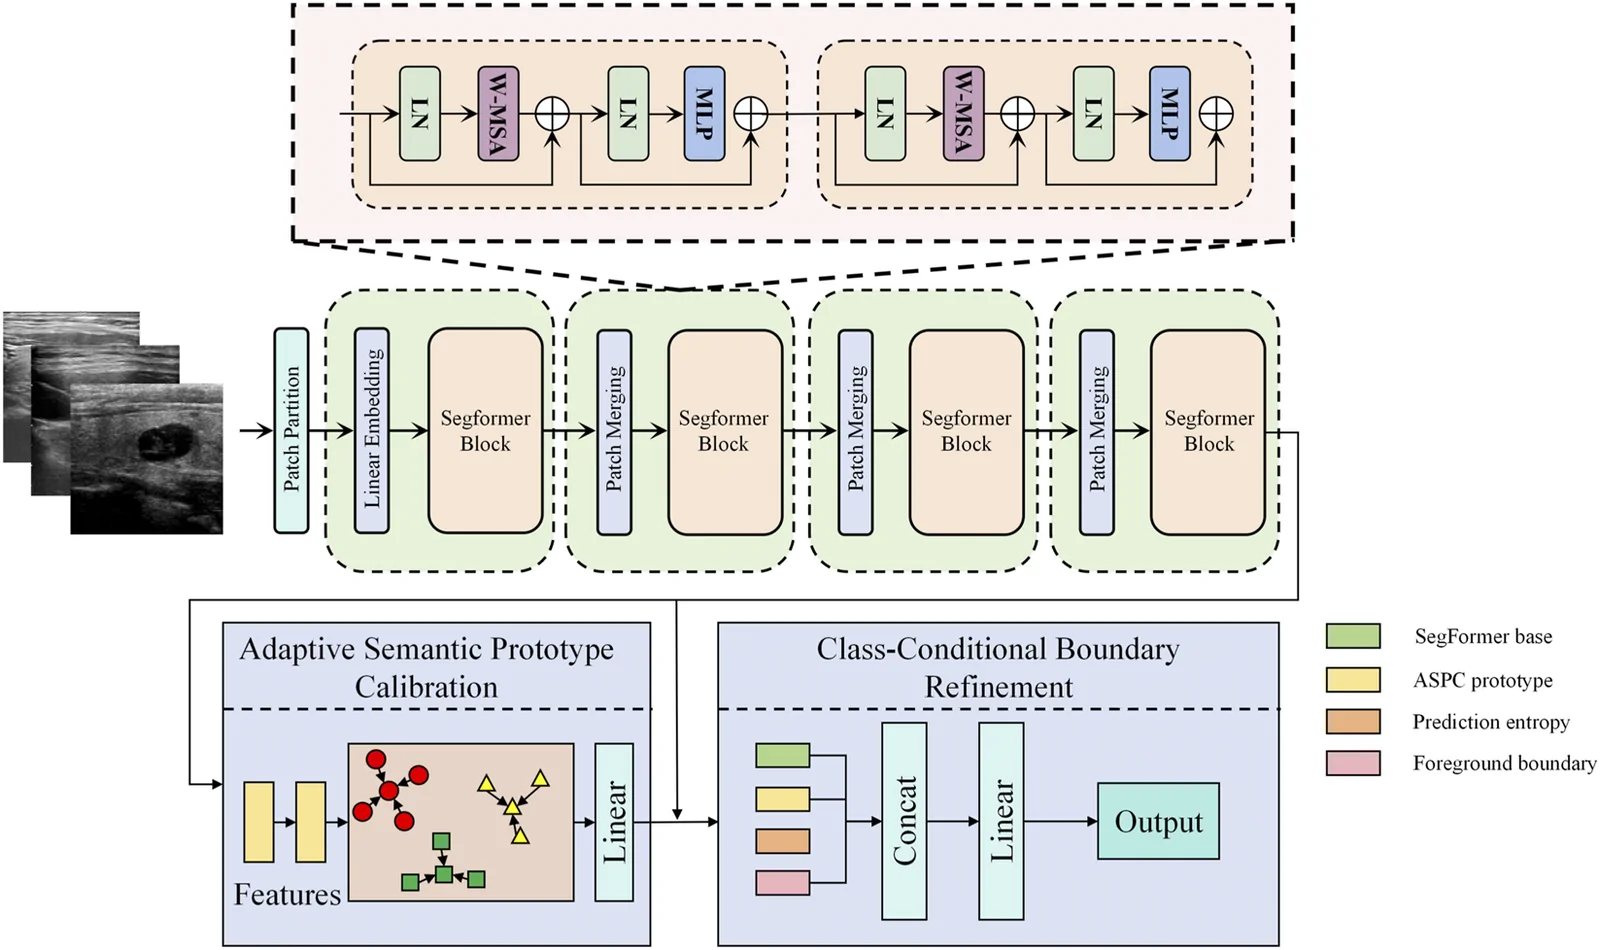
The proposed benign–malignant-aware segmentation framework for thyroid ultrasound images consists of a hierarchical SegFormer encoder, an adaptive semantic prototype calibration module, and a class-conditional boundary refinement module. The model enhances the categorical discriminability of benign and malignant nodule regions through semantic prototypes and further refines ambiguous boundary regions by integrating predictive entropy and foreground boundary responses.
